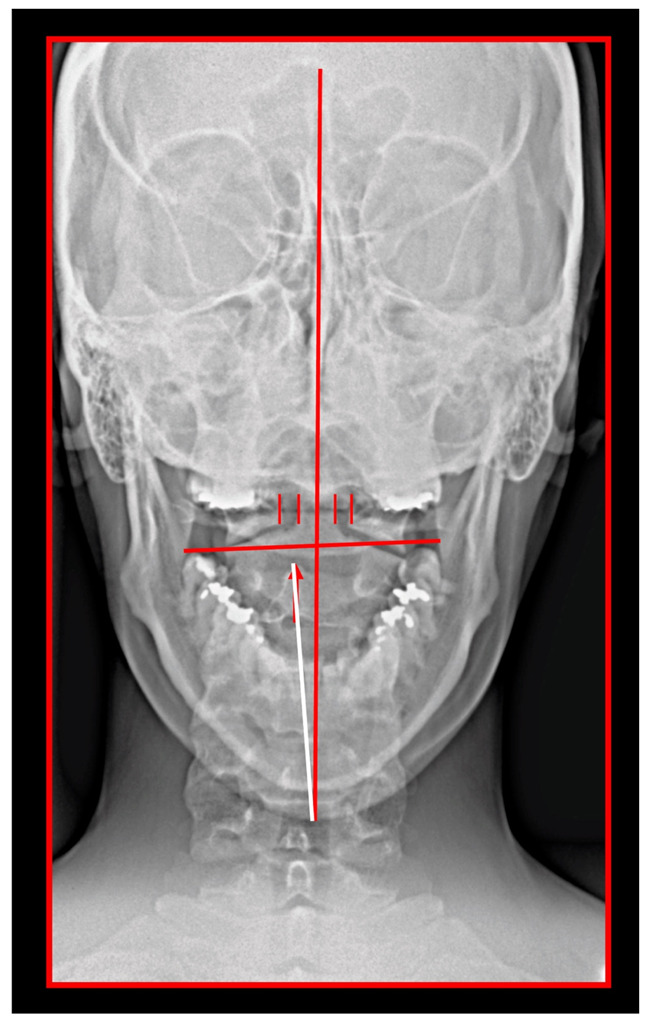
Transoral *atlas*-*axis* X-ray. Skeletal midline—*dens axis*—spinous process of C_2_ should be in one single line. In this patient, spinous process of *axis* is deviated to the right; hence, the *axis* is rotated to the left. The position of the *atlas* is measured from the lateral mass of the *atlas* to the *dens axis*. The space is increased on the right; hence, the *atlas* is rotated to the right. The mandibular occlusal plane is inclined. The cranium is rotated to the left; the left eye pupil is lower than the right.
